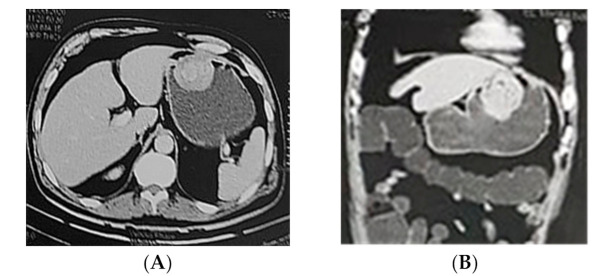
Axial (A) and coronal (B) CT enterocolography showing anterior gastric wall endophytic mass lesion. No outlet obstruction or serosal involvement identified.
